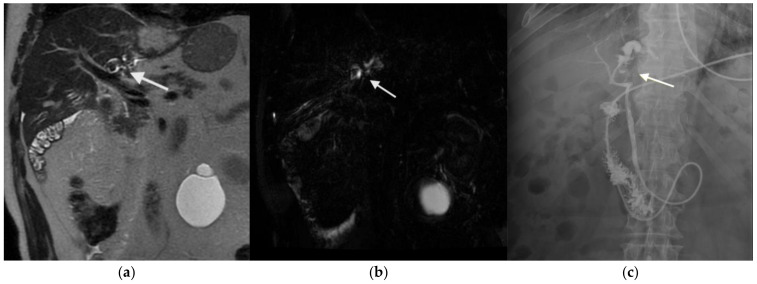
(a,b) Magnetic resonance imaging of a patient with suspected intrabiliary lithiasis, shown in T2-weighted FSE and MRCP sequences. A round, hypointense structure is visible in the proximal segment of the left main biliary duct, consistent with a biliary stone; (c) ERCP a few days after demonstrating the calculus as a filling defect surrounded by contrast medium.
